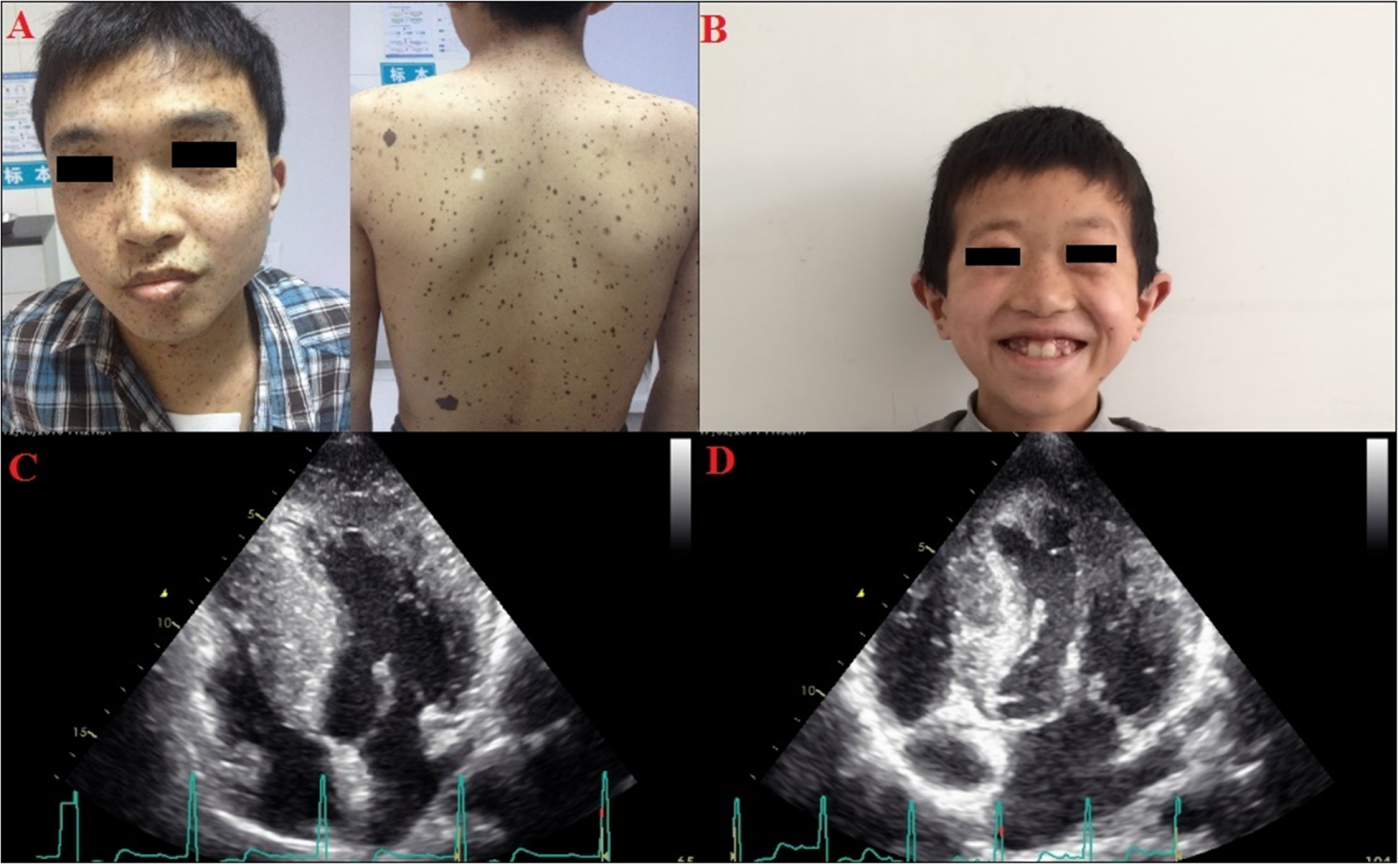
Facial features and echocardiography of two patients with LS. Panel (a)shows vast lentigines in patient LS1. Panel (c) shows the echocardiography of LS1, which reveals heart hypertrophy. Panel (b) shows the facial characteristics of patient LS2. Panel (d) shows the echocardiography of LS2, which also indicates heart hypertrophy
